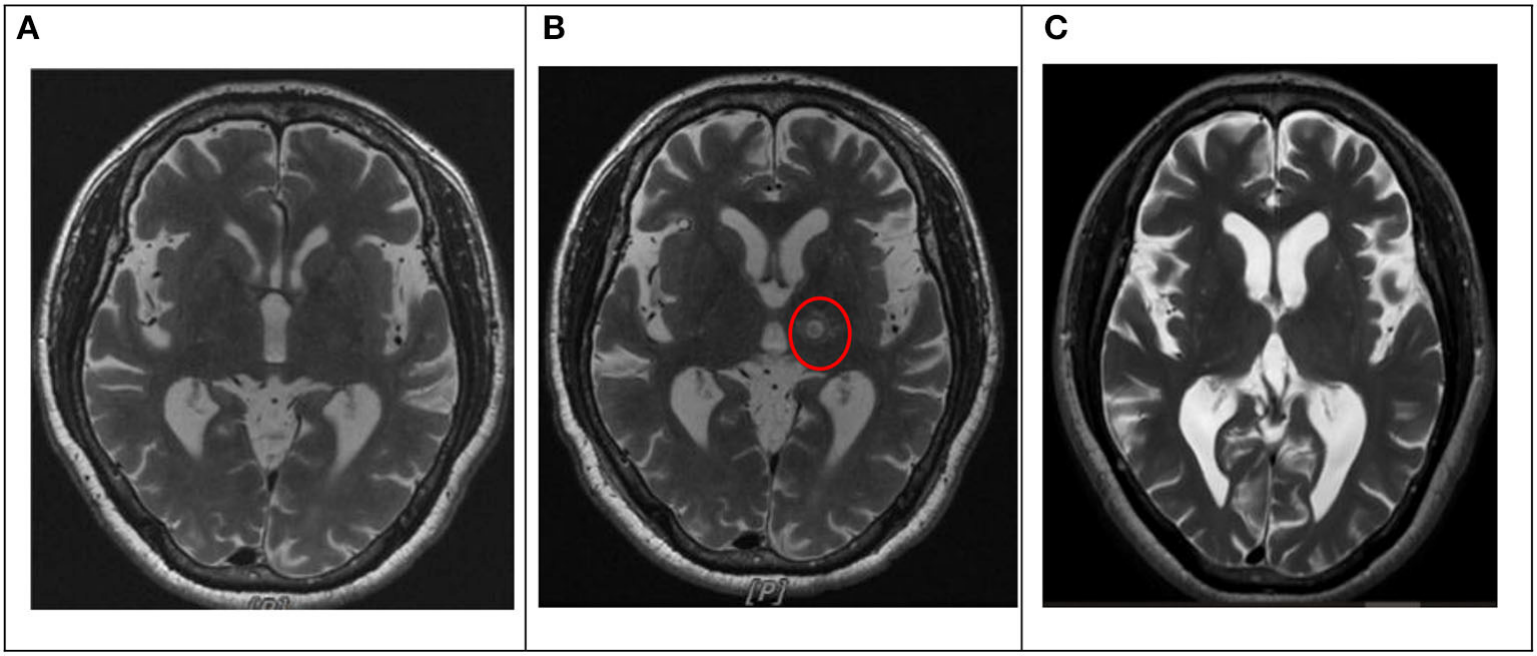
Lesion location on T2-weighted images before and after MRgFUS thalamotomy in one patient. (A) Pre-intervention. (B) Day 1. The target lesion was in the left VIM of the thalamus (red circle). (C) Six months. MRgFUS: magnetic resonance-guided focused ultrasound. VIM: ventral intermediate nucleus.
